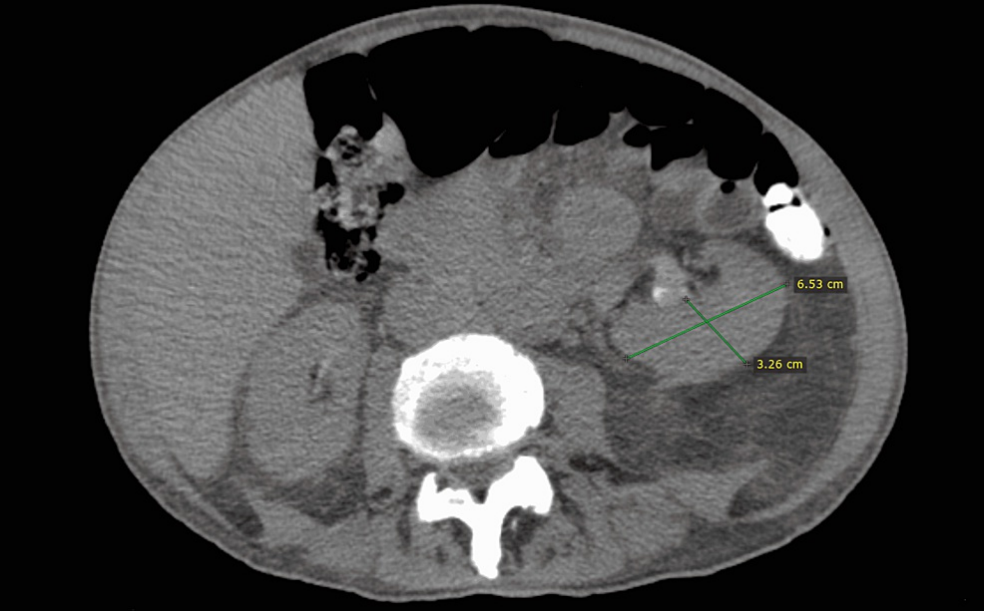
Supernumerary kidney's dimensions Abdominal computed tomography (CT) scan without intravenous contrast medium. The dimensions of the supernumerary kidney are highlighted.
